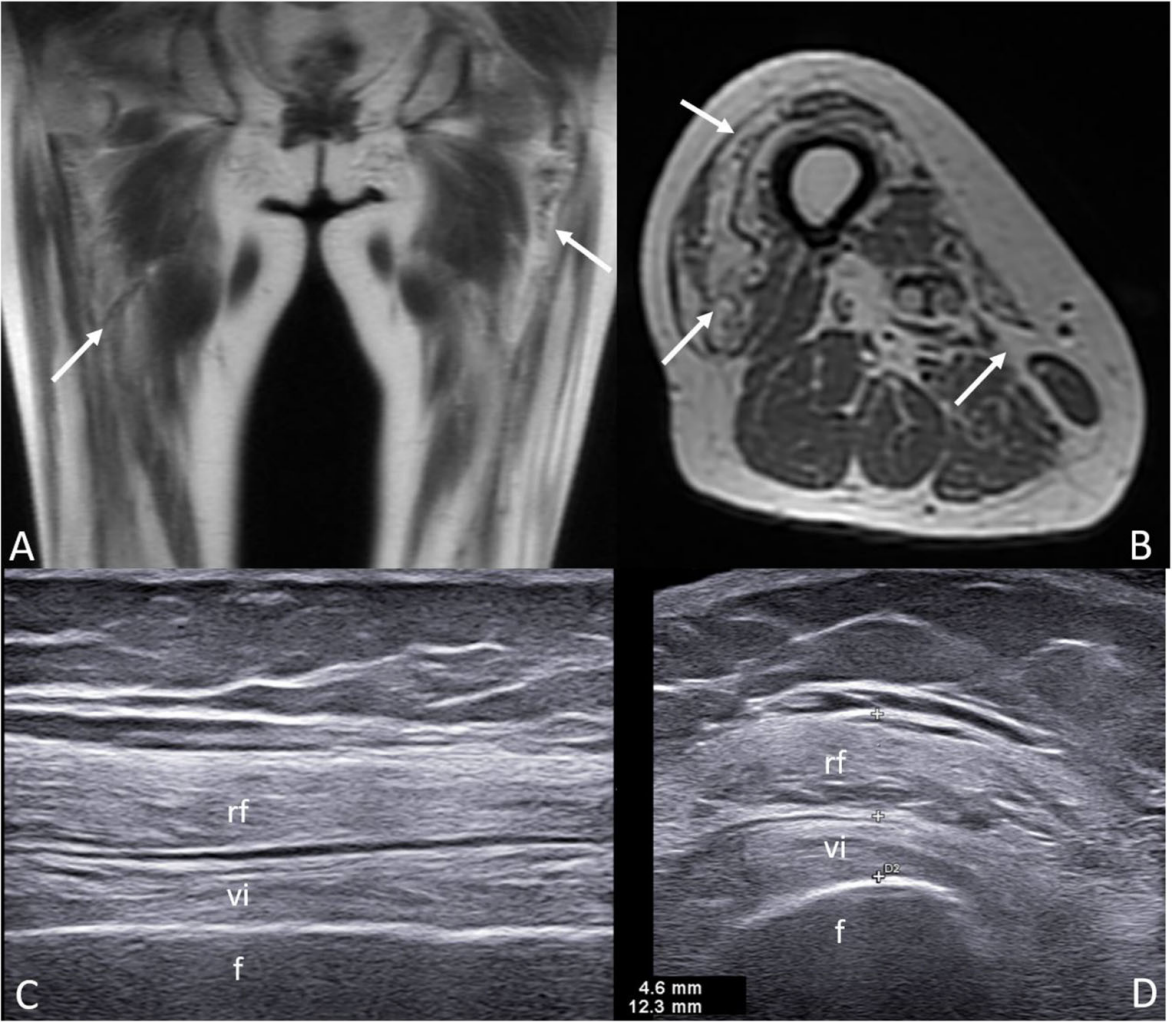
Chronic myositis in a SLE patient. Magnetic resonance imaging shows muscle reduction with advanced fatty infiltration (arrows) of quadriceps muscle bulk on unenhanced coronal (A) and axial (B) T1-weighted images. Ultrasound longitudinal (C) and transverse (D) scans obtained with a 3–11 MHz probe at the anterior thigh level show severely reduced thickness of rectus femoris (rf) and vastus intermedius (vi) muscles. The muscles bellies show a markedly increased echogenicity due to fatty replacement. f = femur
